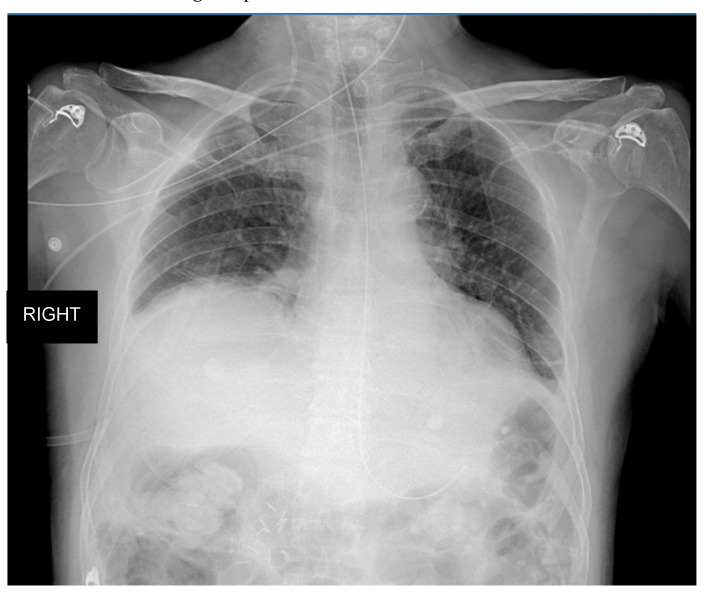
Chest X-ray performed in our unit after clinical worsening of the patient, which documented the presence of right hemidiaphragm elevation.
